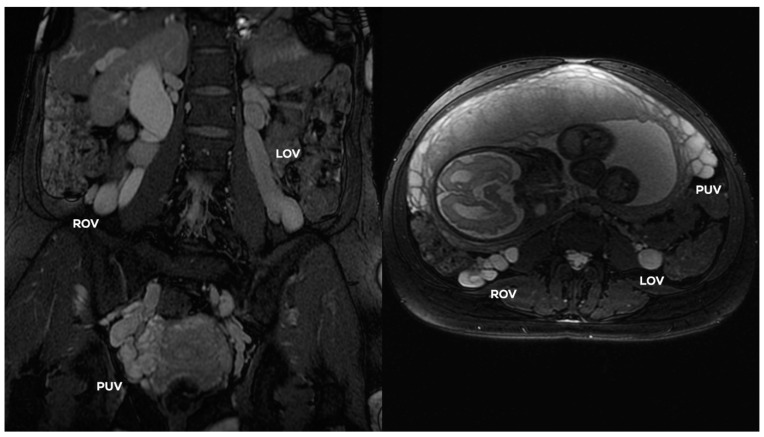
Prenatal MRI. Images in coronal and axial planes. Bilateral ovarian veins distention of 32-year-old woman in third pregnancy. (Courtesy of M. Bekiesinska-Figatowska, PhD; Department of Diagnostic Imaging, Institute of Mather and Child, Warsaw, Poland).
